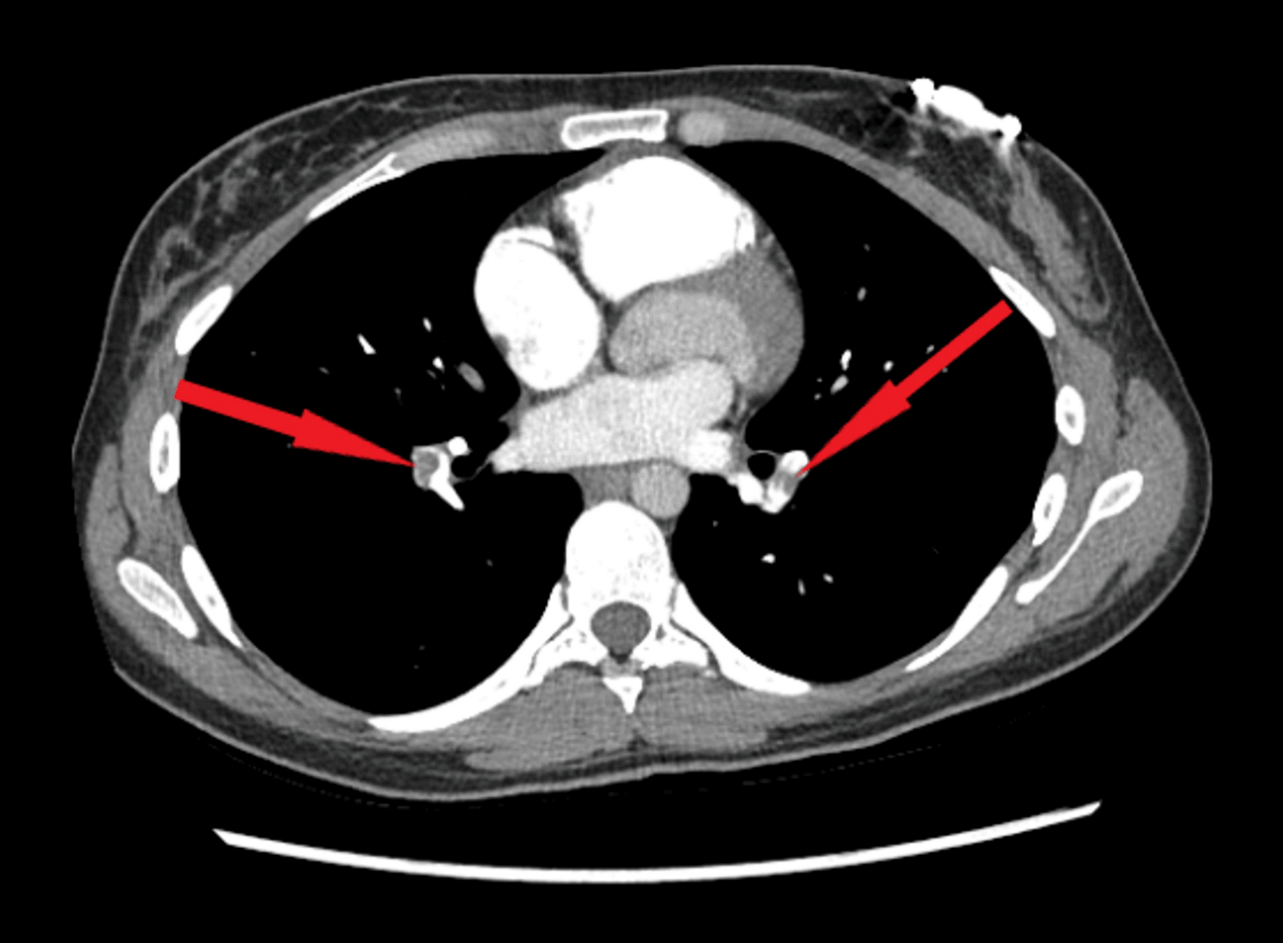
Axial view of CTA of the chest showing bilateral pulmonary embolism, indicated by the red arrows CTA: computed tomography angiography.
